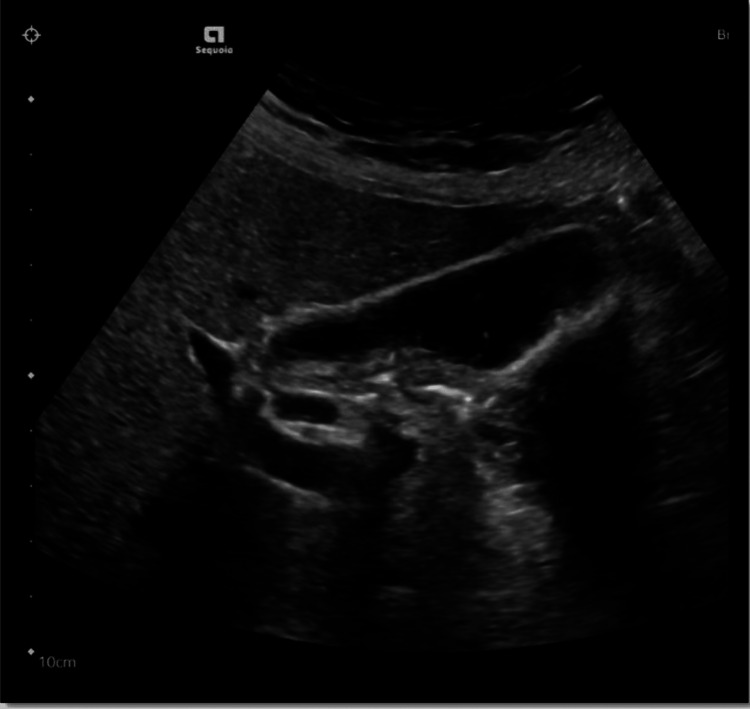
US abdomen: Cholelithiasis. No evidence of acute cholecystitis. US: ultrasound
